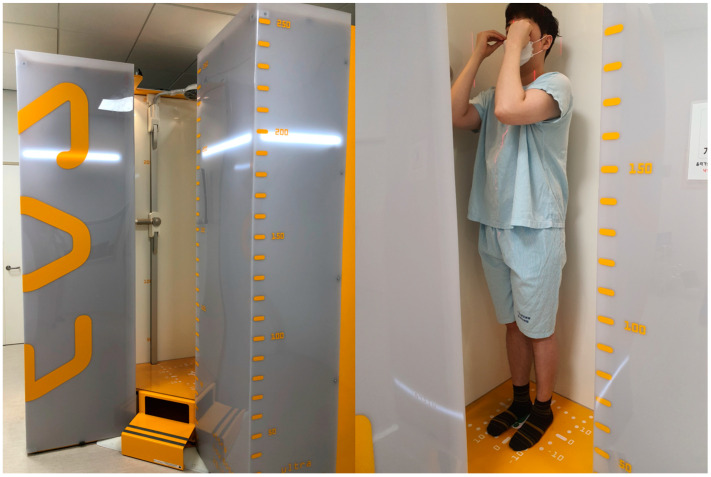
The EOS^®^ imaging system comprises two pairs of X-ray tubes and detectors positioned at 90 degrees to each other. Patients are asked to stand comfortably with their feet side by side, knees straight, and fingertips covering their eyes to prevent the vertebral body from being obscured.
